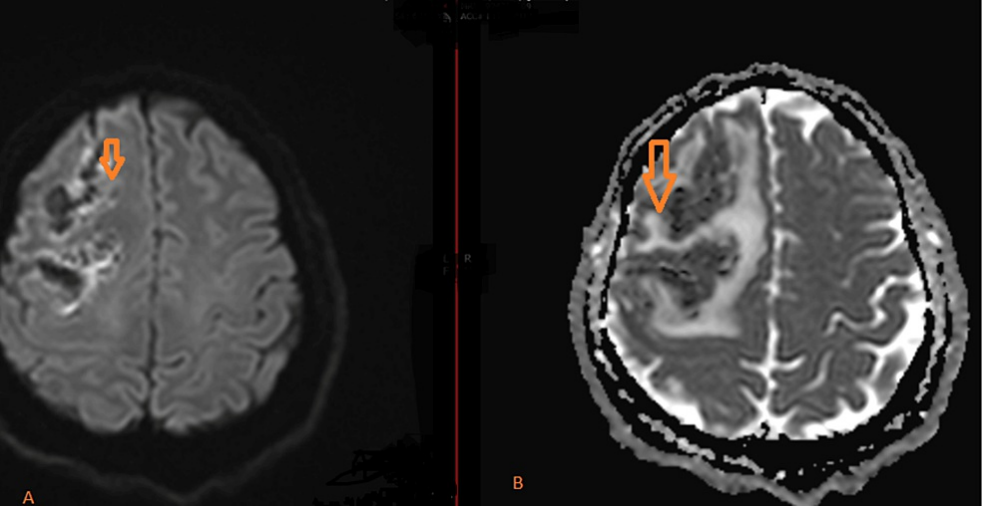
(A) Diffusion-weighted image (DWI) and (B) apparent diffusion coefficient (ADC) map (A) DWI: diffusion restriction at the peripheral edges of the lesion, confirmed by low attenuated signals on the ADC map (B) (arrow).
